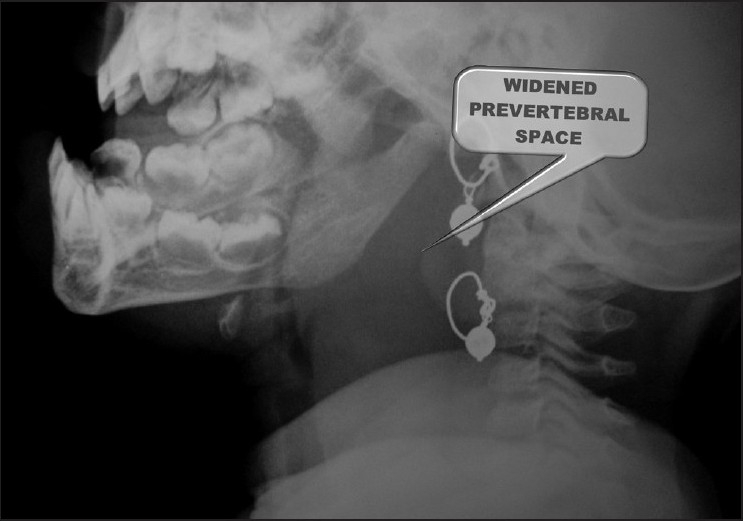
Widened prevertebral soft tissue shadow in X-ray neck lateral view
